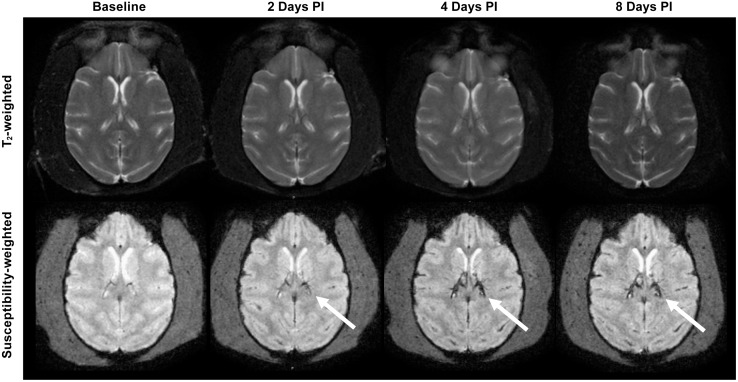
Images of an AGM that underwent MRI before and after aerosol infection with NiV. In this model, neither infection route led to abnormal signal intensity lesions to suggest encephalitis within the brain parenchyma on T2 or on FLAIR weighted images (T2 weighted image, upper row). There was no evidence of abnormal enhancement seen after contrast injection. However, in 2 of the 6 AGMs studied, susceptibility weighted images showed increased visibility/prominence of the deep central and superficial venous structures (white arrows, lower row), compared to baseline, as early as 2 days after infection. This could reflect increased deoxyhemoglobin concentration within the vessels dues to vasodilation and venous stasis possibly part of the systemic manifestations of Nipah virus infection. No parenchymal hemorrhagic changes were seen.
